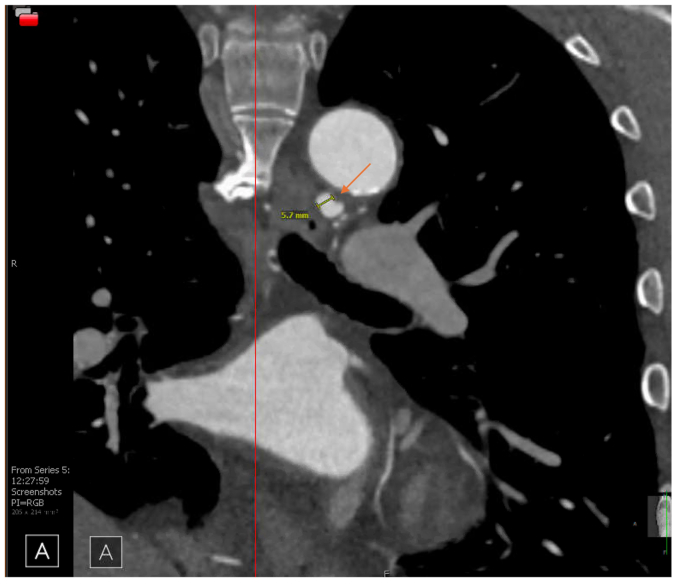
Computed tomography angiogram (CTA) coronal view showing right bronchial artery aneurysm measuring 5.7 mm, *orange arrow*.
